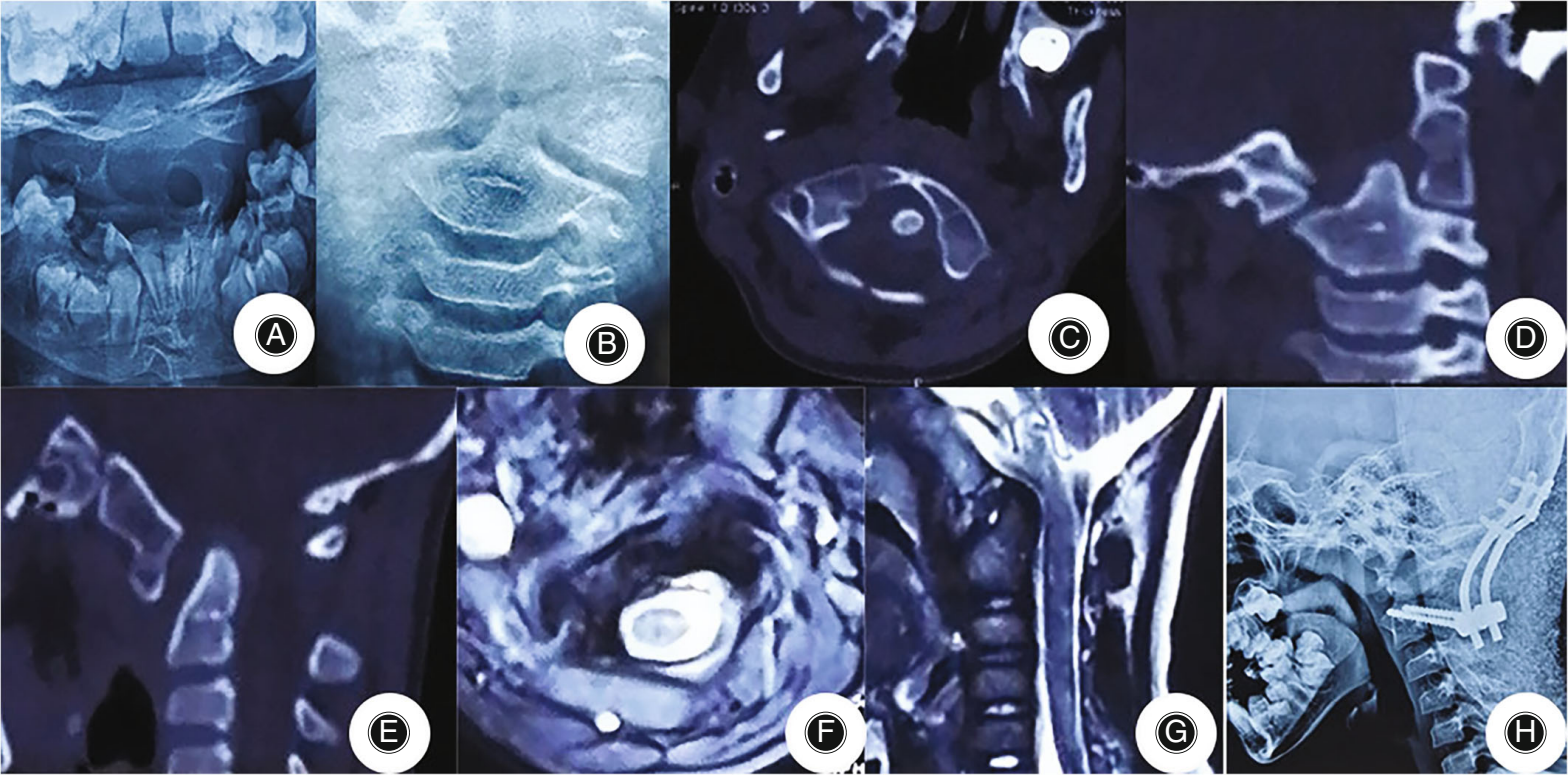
The patient, male, 8 years old. (A) Mouth‐opening position. (B) Anterior position X‐ray films of cervical vertebrae pre operation. (C–E) Preoperative CT images showed rotational fixation of occipital atlantoaxial, and ADI was 4.6mm. (F) Axial MRI indicated relaxation of transverse ligament. (G) Sagittal MRI shows kyphosis of C2 vertebrae and corresponding horizontal spinal canal stenosis. (H) Post operation 7 days, lateral image of the cervical spine showed good reduction and internal fixation and ADI decreased to 2.4 mm.
